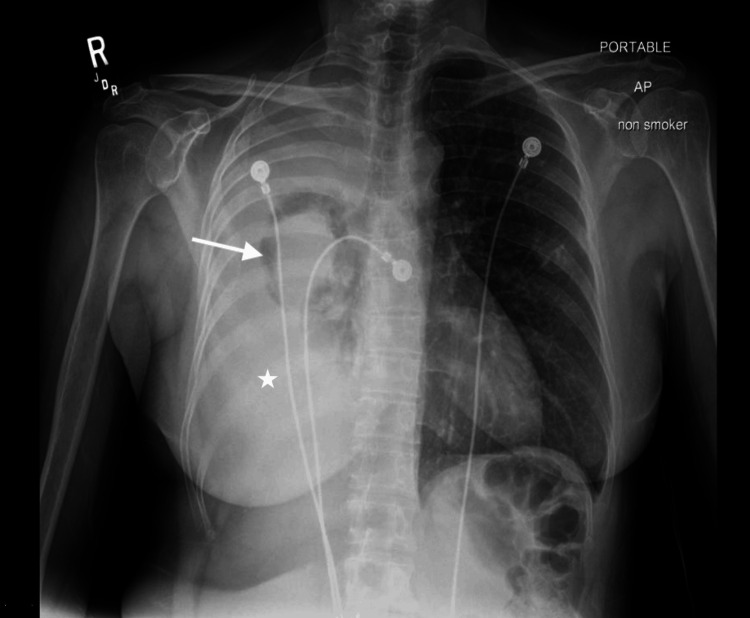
Single front-view chest X-ray: pleural effusion (white asterisk) and cavitary lesion (white arrow)
